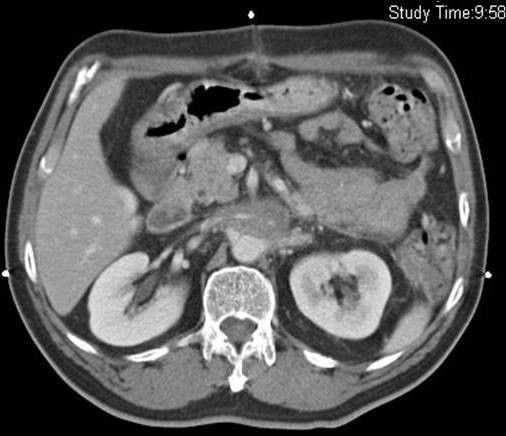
Preoperative abdominal CT scan showing retroperitoneal mass encasing the right renal vein (arrow).
